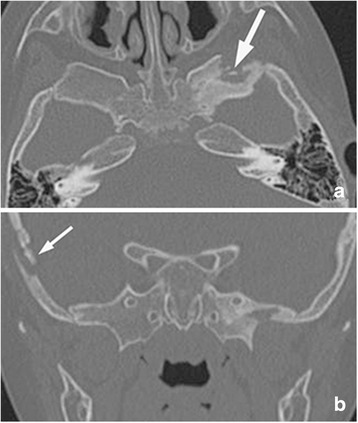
Axial CT shows focal bony erosion of the left greater wing of sphenoid bone with well-defined margin (a), which was pathologically confirmed LCH. Focal well-defined lytic lesion with punched-out appearance of squamous part of the temporal bone is clearly demonstrated on CT (b)
